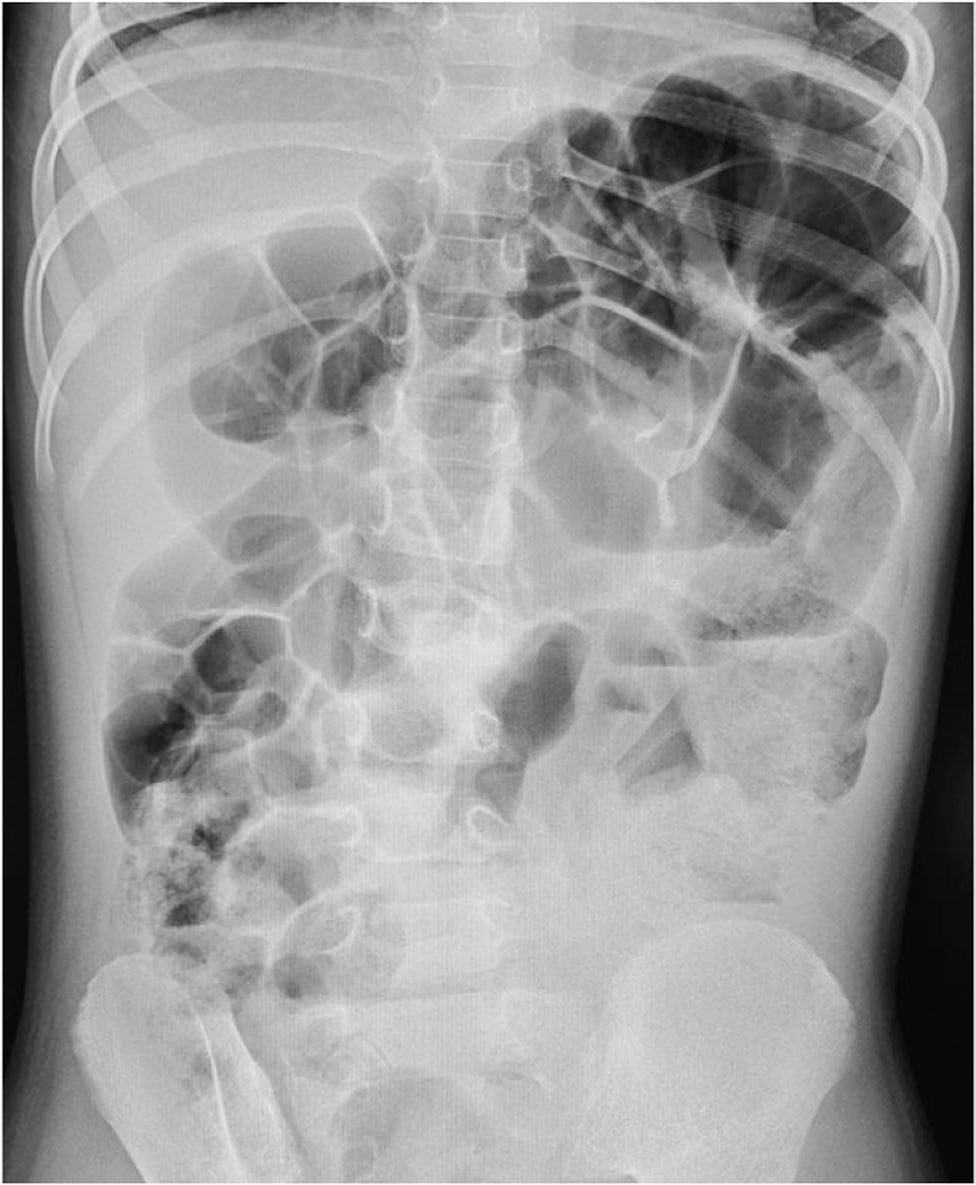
Plain erect abdominal radiograph showed diffuse intestinal gas accumulation
